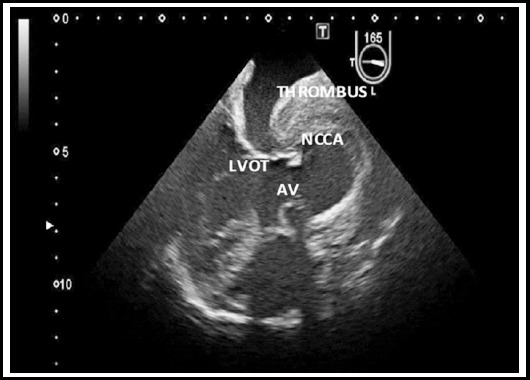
Transesophageal Echocardiography long axis view of aorta showing non-coronary cusp sinus of Valsalva aneurysm [NCCA] and thrombus (AV = Aortic Valve, LVOT = Left Ventricle Out flow Tract).
